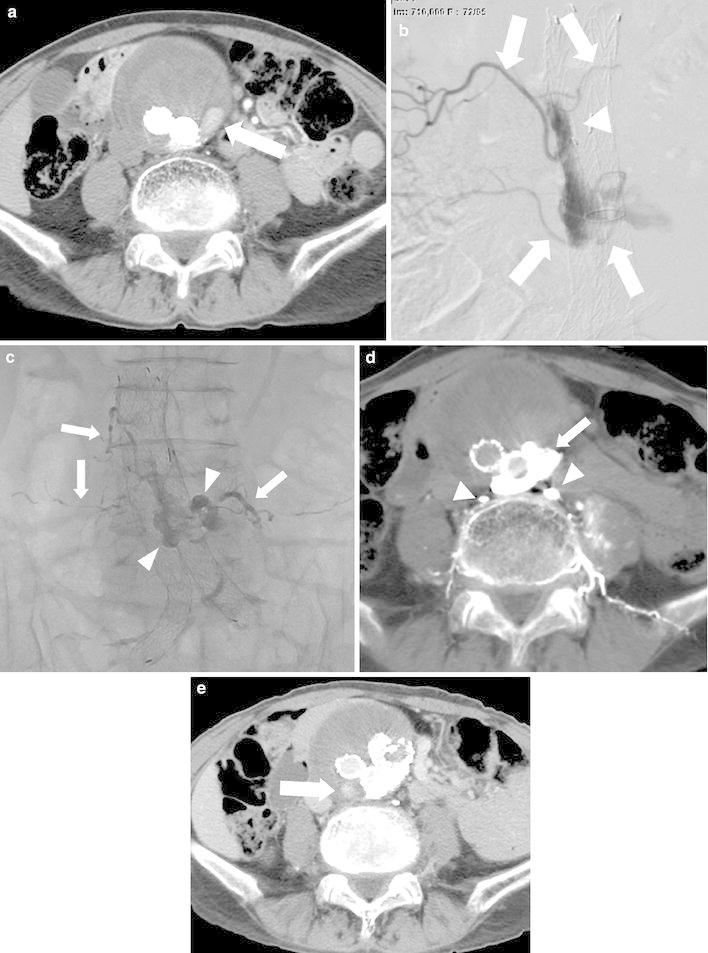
Images of an 88-year-old man with T2EL (case no 1, Table 1). a Enhanced CT showed that the aneurysm expanded with persistence of T2EL (*arrow*) 15 months after EVAR. b Endoleak angiogram performed via a microcatheter demonstrated that the endoleak involved four vessels (*arrows*). *Arrowhead* indicates the micro catheter tip that was advanced into the endoleak sac through the left fourth lumbar artery. c Roentgenogram showed NBCA injected to fill simultaneously the aneurysmal sac (*arrowheads*) and the involved lumbar arteries (*arrows*). d Plain CT showed radiopaque NBCA accumulations in the endoleak sac (*arrow*) and lumbar arteries (*arrowheads*). e Enhanced CT obtained 6 months after the embolization showed another small endoleak (*arrow*) with no further expansion of the aneurysm
